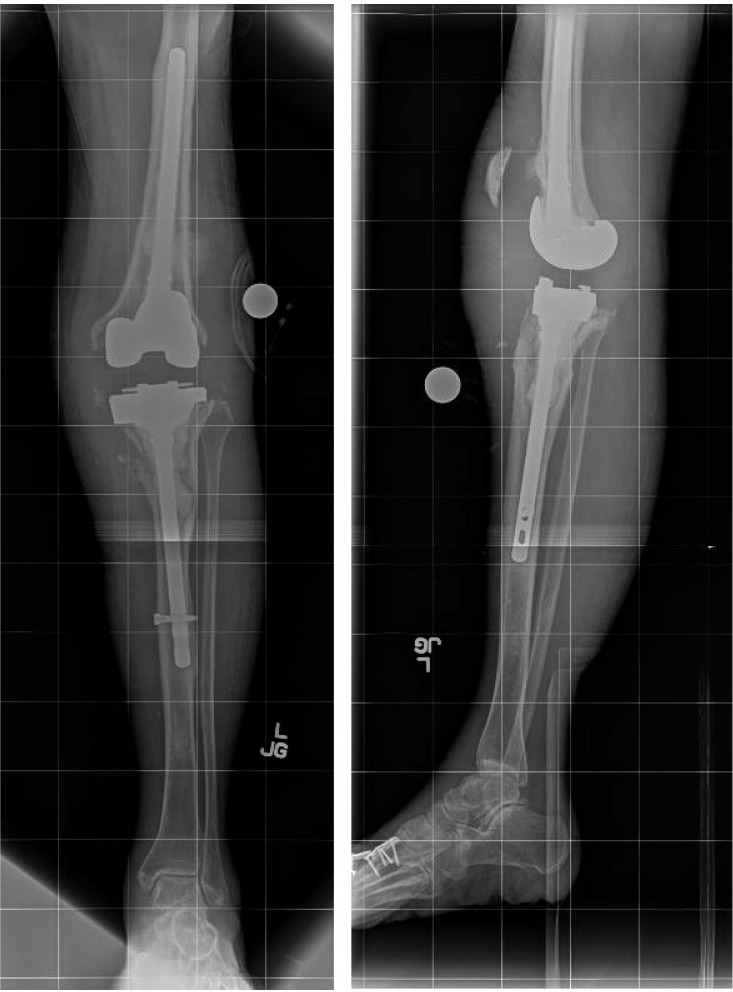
8/5/2014. AP (A) and Lateral (B) radiographs demonstrating substantial effusion of the knee joint, and lucency and periprosthetic loosening around the stemmed tibial implant.
